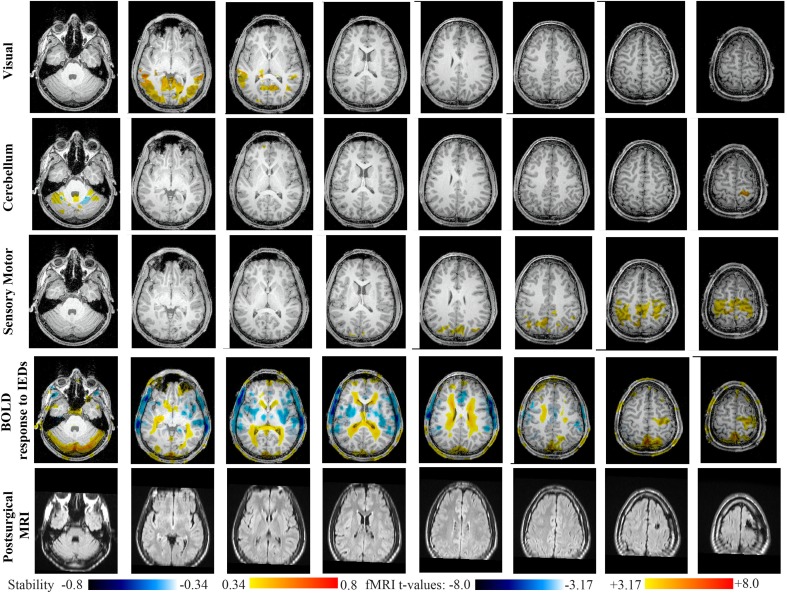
Evaluation of DANI on Patient 6 with right frontal FCD. Results are presenting most salient stability changes observed in *Cmap*_*n*_ followed by a *t*-map of the BOLD response to epileptic discharges and a postsurgical FLAIR MRI, all resampled in the native anatomical MRI space of the patient. Most salient stability changes observed for *Cmap*_*n*_ of Visual, Cerebellum, and Sensory Motor networks are presented. The Visual and Cerebellum networks were also involved in significant interactions of modularity changes at *p* < 0.001. Same colormap conventions than in Figure 6.
